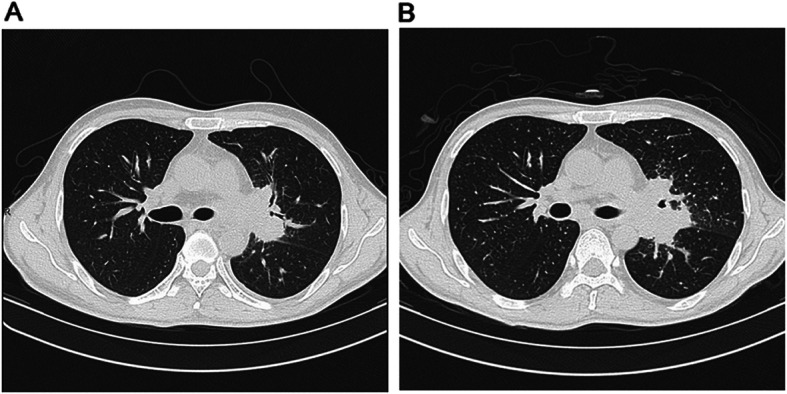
Contrast-enhanced CT images of an TB-IRIS patient experiencing paradoxical TB-IRIS. a. A man was diagnosed as lymphatic tuberculosis at the time of HIV diagnosis. No obvious abnormal findings were recorded in the lung fields. b. ART was intiated after 14 days of anti-TB treatment. After 28 days of ART, he had worsening lymph node enlargement and military infiltration of the lungs
